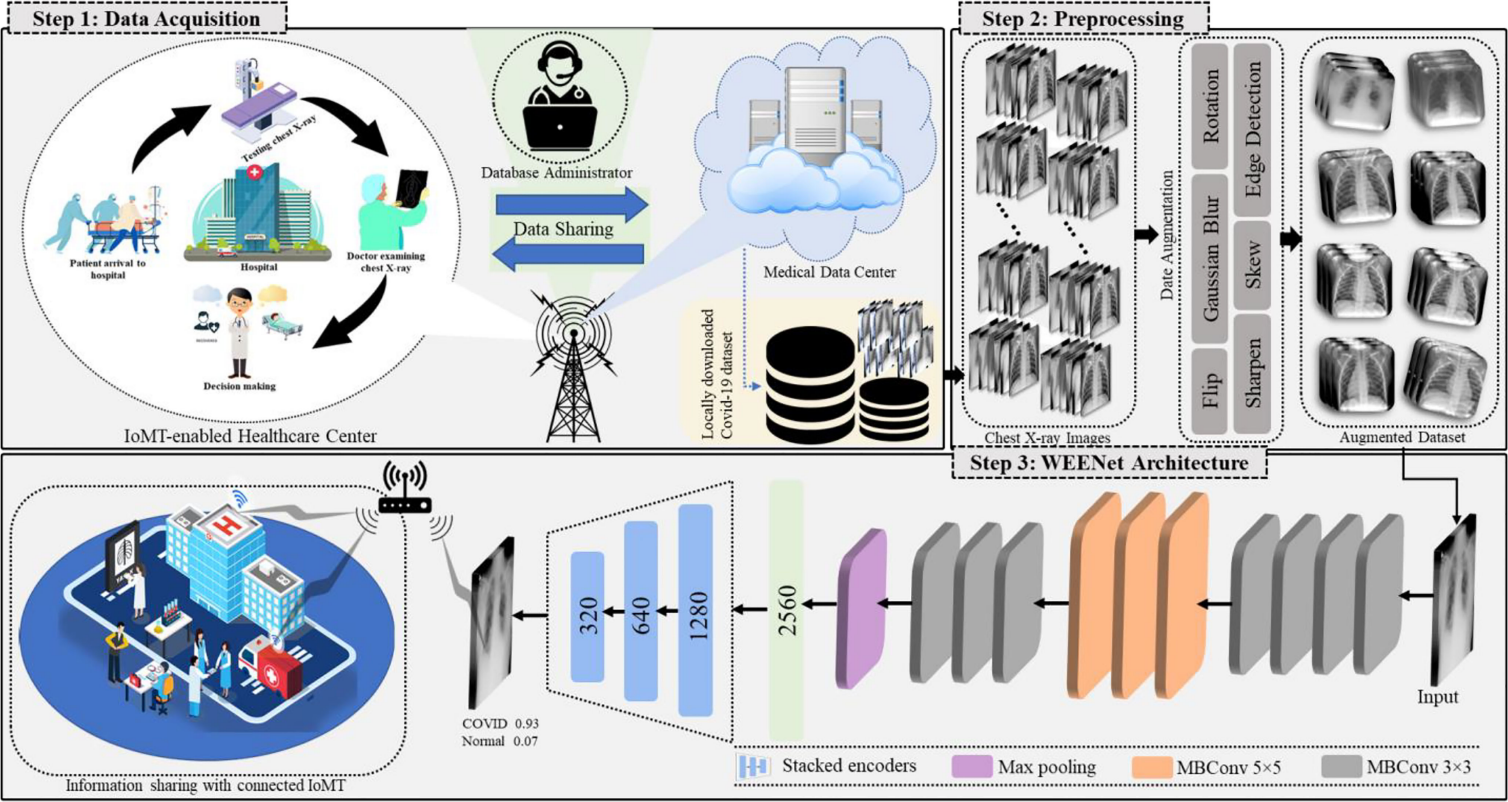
Overview of the proposed WEENet-assisted framework for COVID-19 diagnosis using chest x-ray images with the support of 5G technology and efficient management for IoMT environments.
